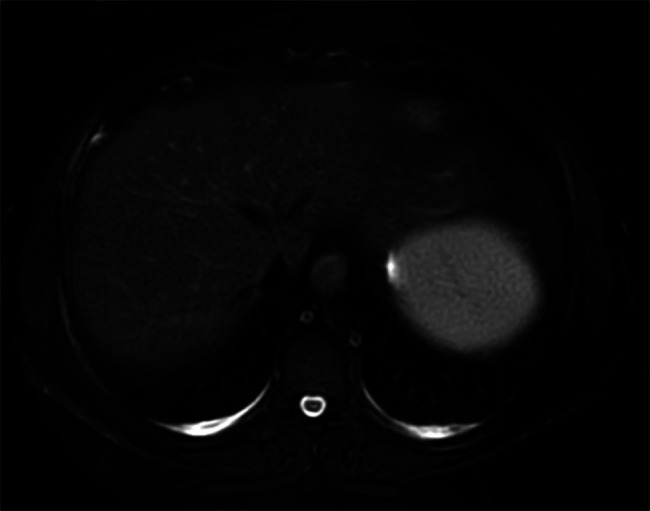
T2 FLAIR-weighted magnetic resonance imaging demonstrating resolution of hepatic granuloma after treatment with certolizumab pegol. FLAIR, fluid-attenuated inversion recovery.
